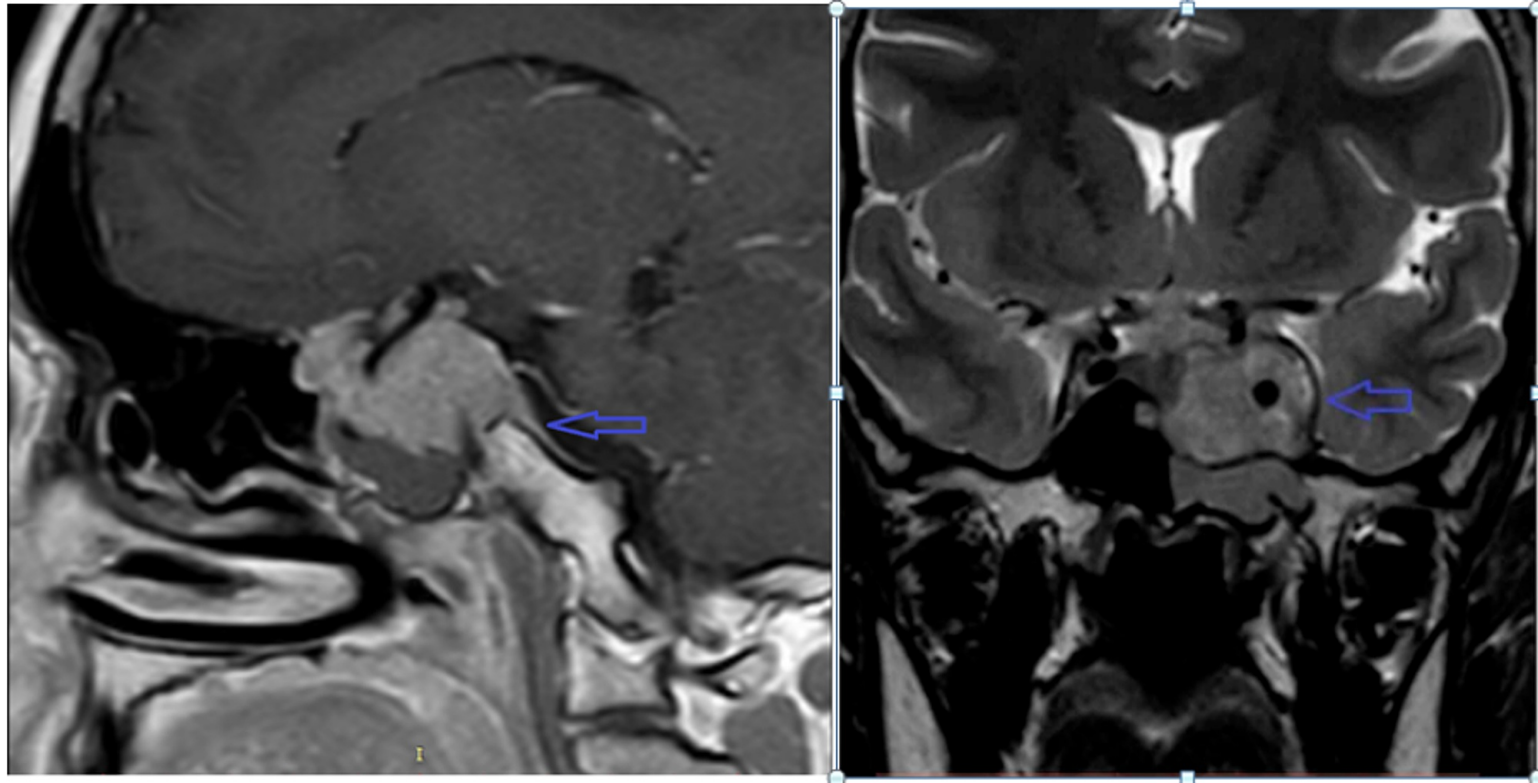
Post-contrast T1 and coronal T2-weighted sequences show a large lobulated parasellar and sellar mass showing avid enhancement. There is the encasement of the left internal carotid artery, with dural tail along the left lateral aspect. Also note the enhancing mass involving the optic chiasm (blue arrows).
